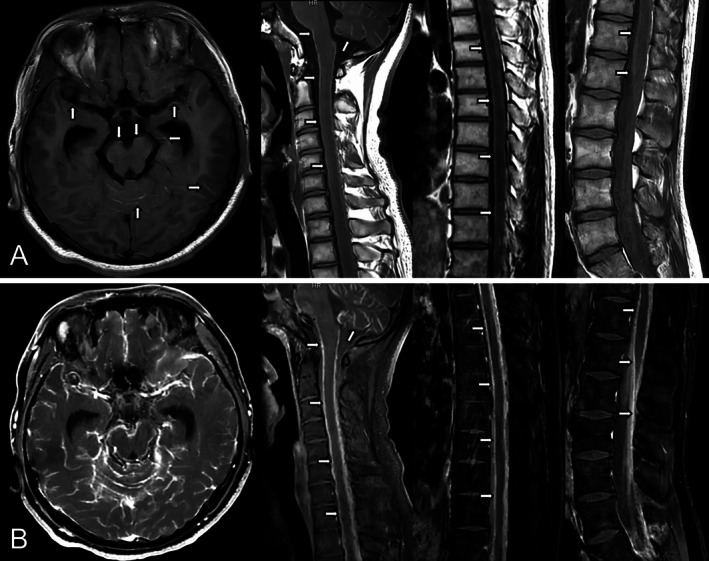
MRI brain and spine. (A) Non‐contrast T1‐weighted images show hyperintensities along sulcal spaces and the surface of the spinal cord (arrows). (B) Post‐contrast T1‐weighted images show diffuse irregular and continuous leptomeningeal enhancement, without any skip area from the cerebral hemispheres to the conus medullaris (arrows). Hydrocephalus is revealed.
